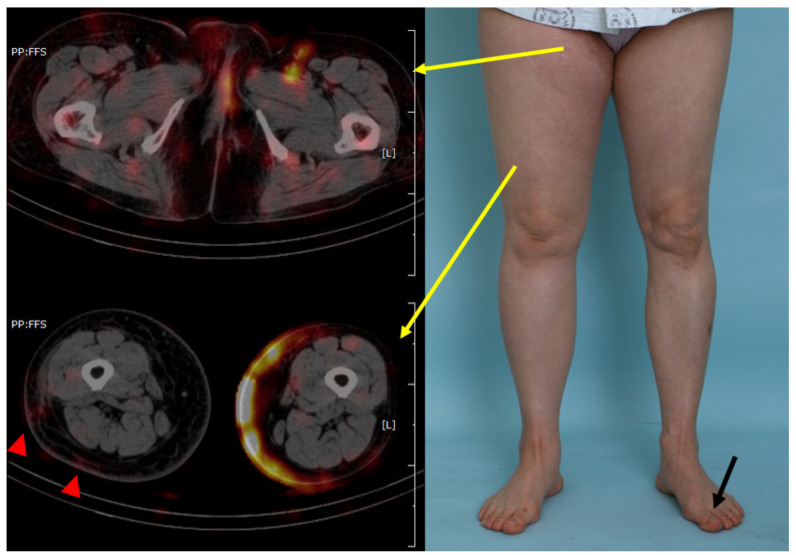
Comparison of the clinical photograph and SPECT scan images of a 55-year-old woman with secondary right-sided lower-extremity lymphedema following cervical cancer treatment with pelvic lymph node dissection. The patient had a disease duration of 9 years. The red area denotes the isotope distribution in the tissue. Radiological findings clearly demonstrate pelvic retention and reverse lymph flow. Yellow arrows indicate the corresponding locations of the SPECT-CT image slices, with the arrow starting points marking the slice level. Red arrowheads denote areas of dermal diffusion, while the radioisotope was injected only into the healthy foot (black arrow).
